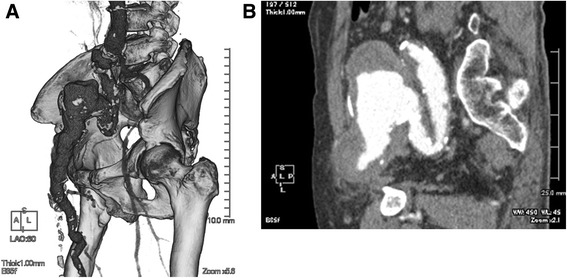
Preoperative contrast-enhanced abdominal computed tomography revealed the presence of an irregular aneurysm from the right common iliac artery to the right common femoral artery, the maximum diameter of which was 50 mm (a left anterior oblique view in three-dimensional computed tomography; b sagittal view)
